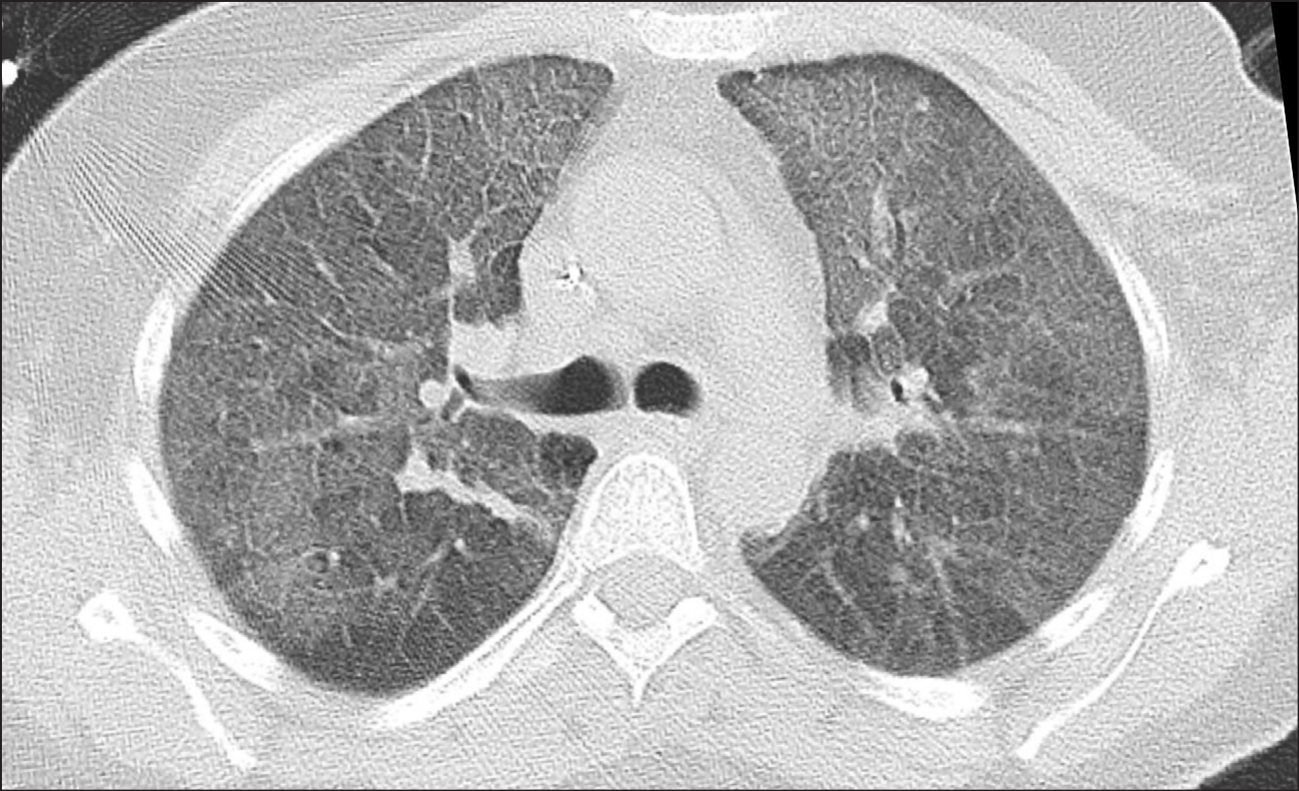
Chest HRCT showing crazy-paving pattern, and a partially imaged consolidation in the right upper lobe, with no pleural effusion in a patient with ARDS (same patient as in Figure 2).
